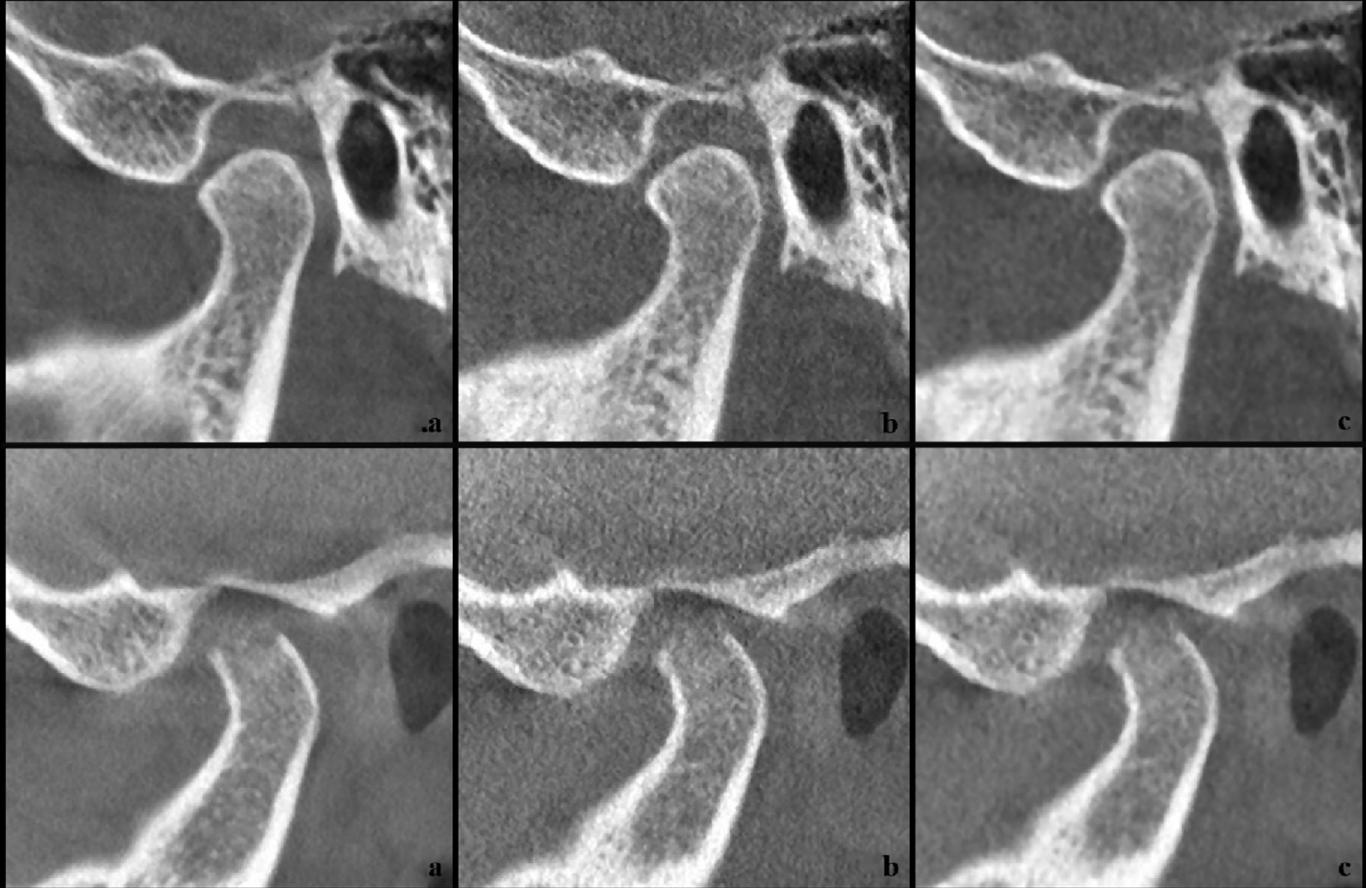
Examples of cone beam CT images for two temporomandibular joints using the three protocols (a) default, manufacturer recommended, (b) low-dose and (c) processed low-dose. 1 (a, b, c): case with no signs of degenerative joint disease; 2 (a, b, c) case with signs of degenerative joint disease.
